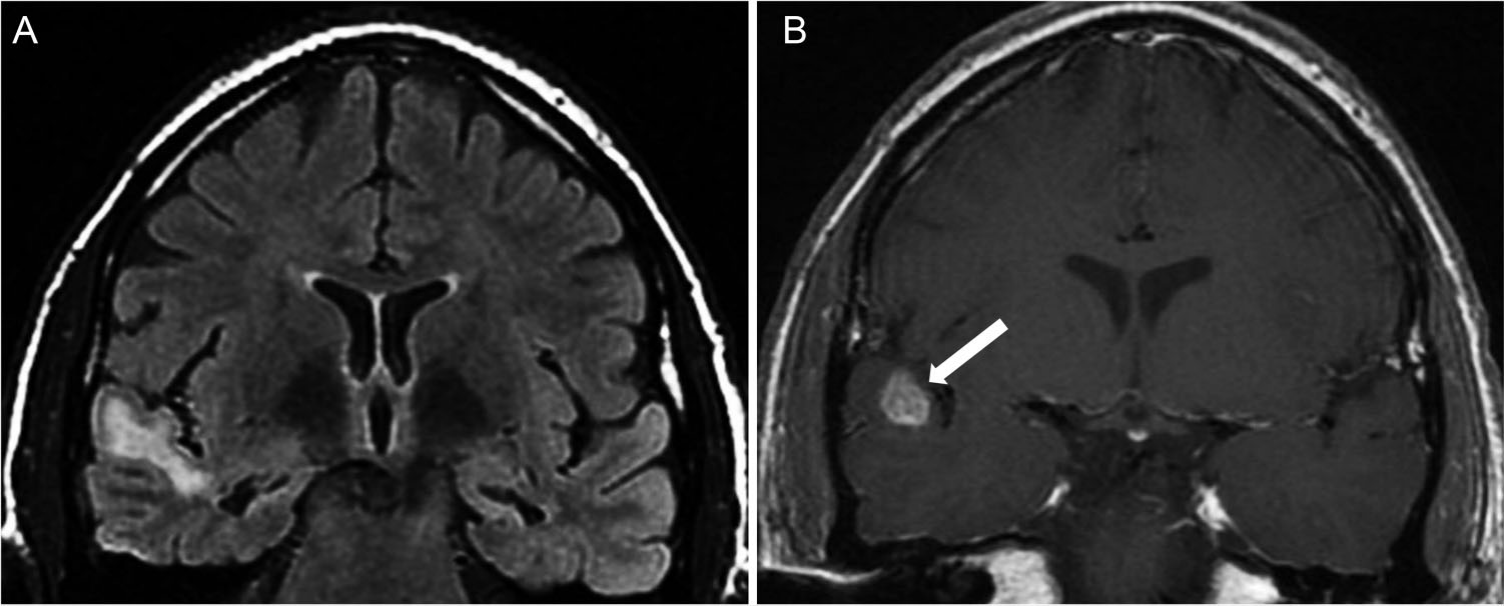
Bilateral hippocampal sclerosis and “dual pathology” in a 34-year-old woman. A) Coronal FLAIR image showing bilateral hippocampal atrophy and hyperintensity as well as a hyperintense lesion in the right superior temporal gyrus. B) Coronal T1-weighted image after a contrast administration revealed a nodular mass enhancement situated at the gray-matter-white-matter junction (white arrows); this mass might correspond to a developmental neoplasm. This lesion appeared stable on consecutive MRI scans.
